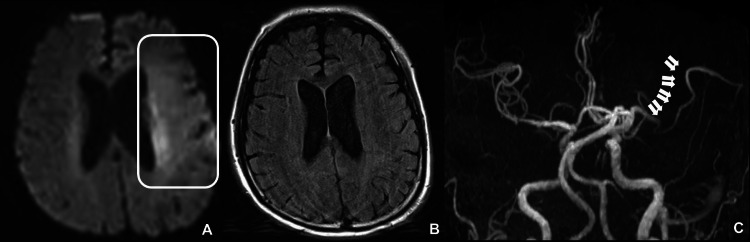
Initial MRI and MRA findings at admission (A) DWI reveals a faint high-signal area in the region of the left MCA (white box). (B) No signal changes are observed in the same area on FLAIR. (C) MRA shows occlusion of the left MCA (white arrows). MRI, Magnetic resonance imaging; MRA, Magnetic resonance angiography; DWI, Diffusion-weighted imaging; MCA, Middle cerebral artery; FLAIR, Fluid-attenuated inversion recovery
